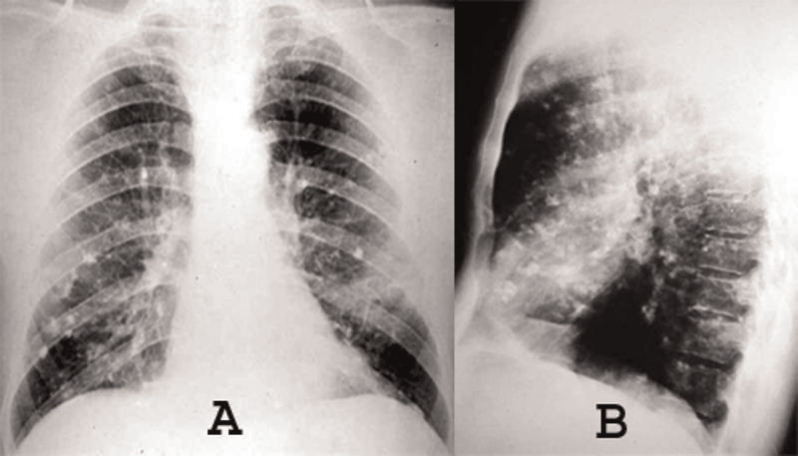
Posteroanterior (A) and lateral chest radiograph (B), performed six years earlier already showed the presence of calcified nodules, which were smaller than in recent radiographs.
